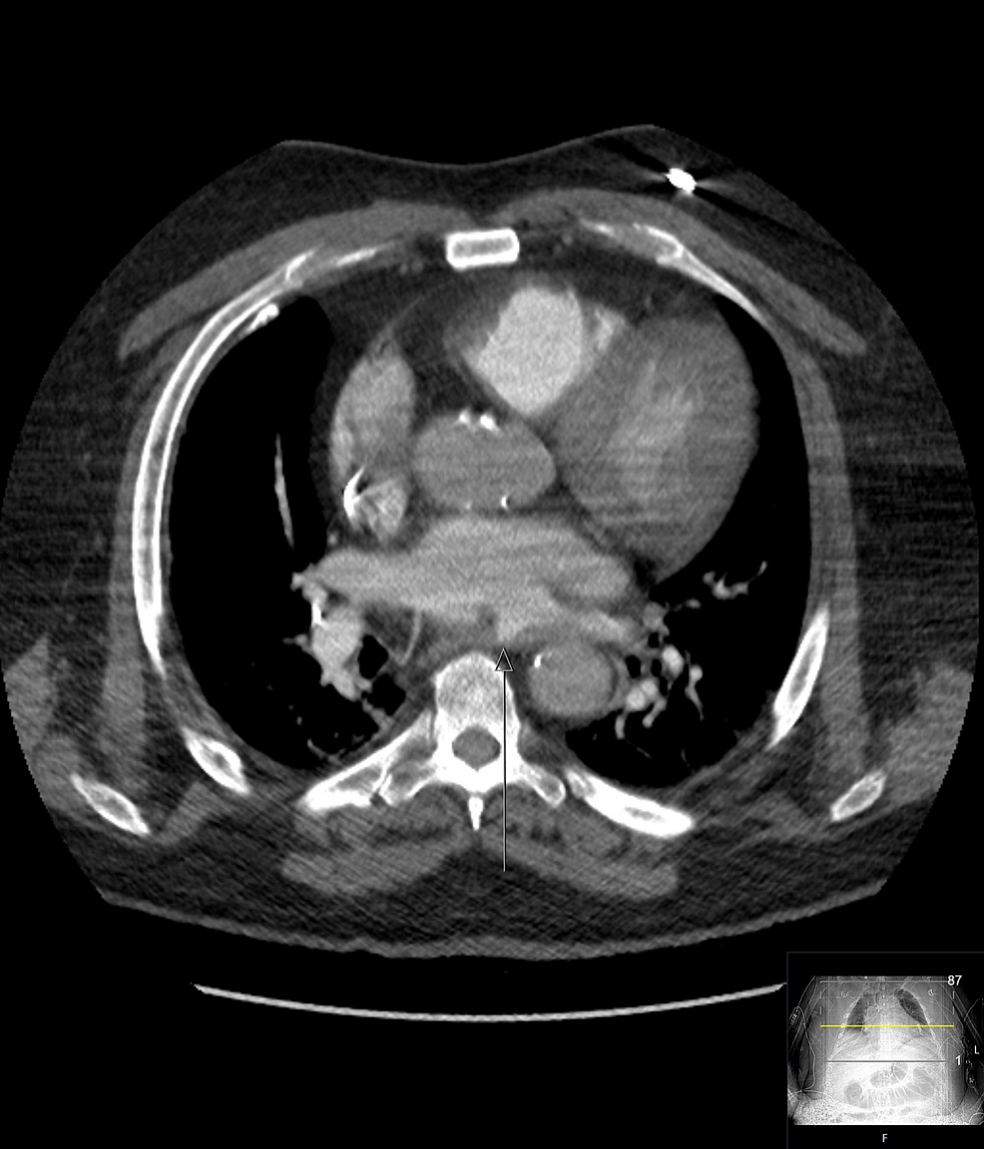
Unusual outpouching of contrast along the posterior aspect of the left atrium directly adjacent to the esophageal lumen. The arrow shows the location of the fistula diagnosed on CT angiography. CT angiography axial chest. CT: computed tomography.
